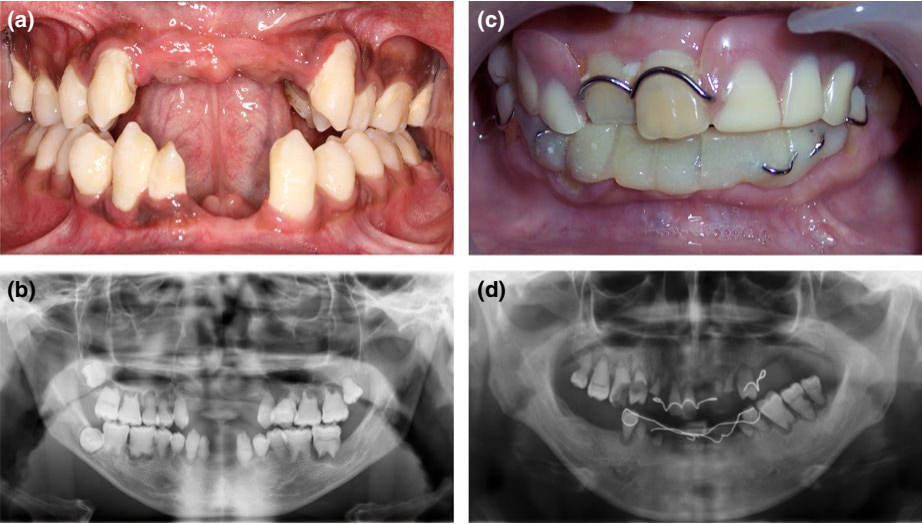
Clinical features of the patients. (a) Intraoral photo shows taurodontic teeth. (b) Panoramic radiograph revealed rootless teeth and periapical cysts. (c) A patient with normal crowns. (d) Panoramic radiograph revealed deformed root with unexplained apices
